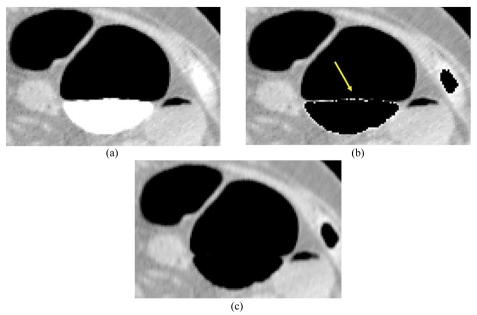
Result of electronic cleansing to an axial image of CT colonography. (Display condition is as follows: thresholding level 160HU, Window Center -200HU, and Window Width 650HU) (a) CTC before EC, (b) Result of segmentation by thresholding and (c) Result of EC using the authors' developed method.
